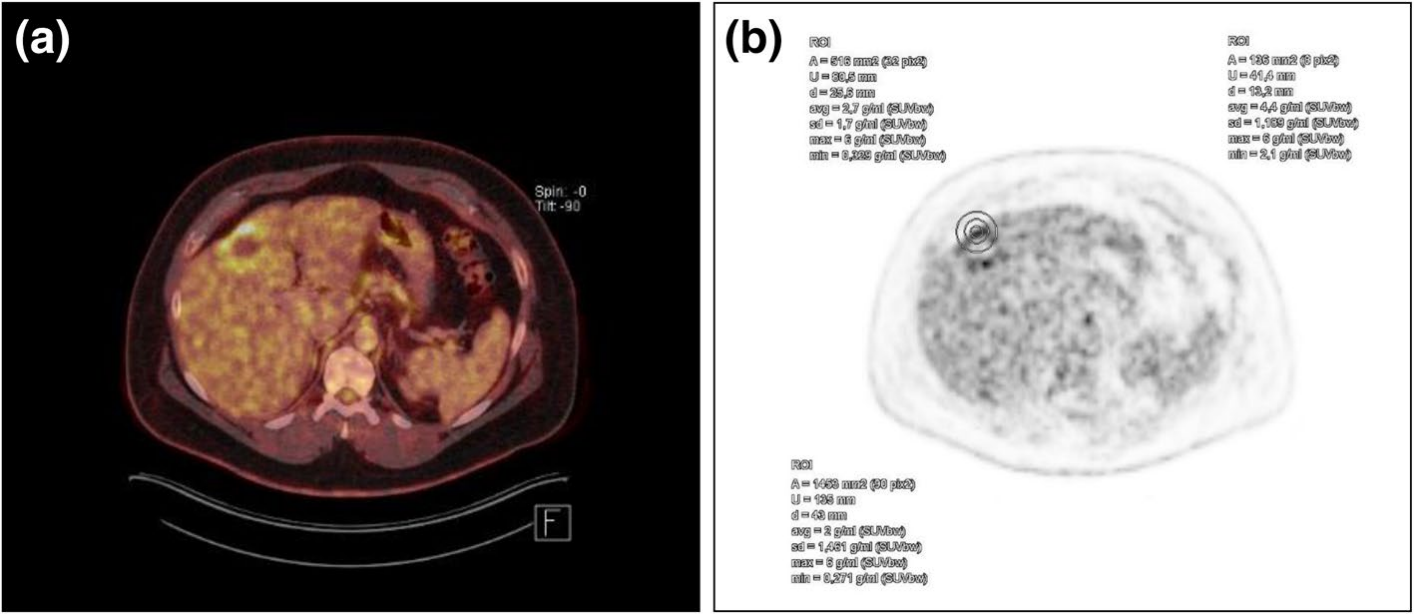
SUVmax is independent of the ROI size, and the measurement is not subject to a partial volume effect, because it is a measurement of punctate signals within the ROI. (a) An active hepatic alveolar echinococcosis lesion (EMUC-CT type II), (b) the same SUVmax was measured for different ROI sizes (indicated with concentric circles).
